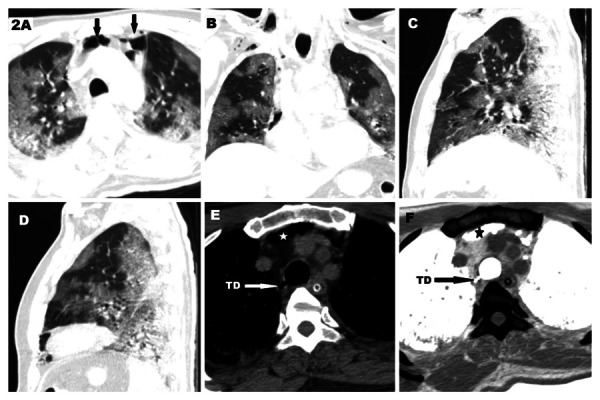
CT scan of a 54-year-old male with severe Covid 19 pneumonia. A. axial CT scan in lung window showing multiple peripheral ground glassing with pneumomediastinum (arrows) . B–D. Coronal, Sagital of right lung and Sagittal of left lung respectively showing peripheral ground glassings typical of covid 19 pneumonia. E. Axial CT scan in mediastinal window showing tracheal diverticulum (TD) and pneumomediastinum (star). F. Inverted CT image of the same showing better depiction of the diverticulum (TD).
